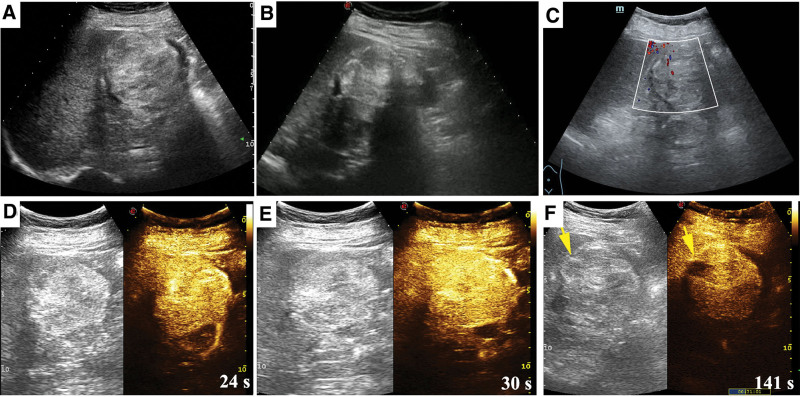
Ultrasonography images of the patient. B-mode ultrasonography revealed an ill-defined hyperechoic mass in the right kidney, with uneven internal echoes (A) and calcification (B). (C) Star-/dot-shaped blood flow detected inside the tumor. Contrast-enhanced ultrasound results showed a “fast in and slow out” pattern in the tumor (D–F) and unenhanced area (F).
